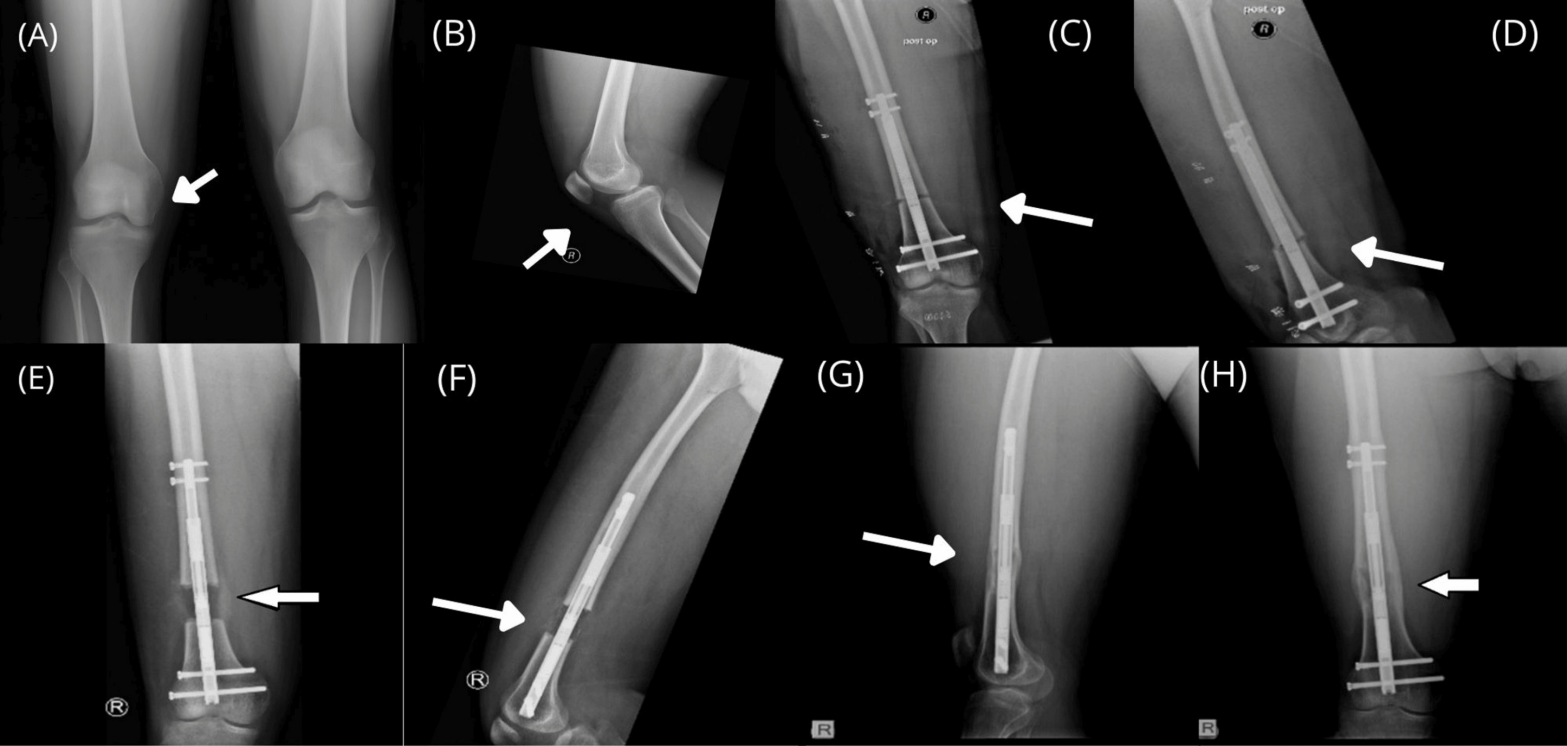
Radiographs Radiographs of a 24-year-old patient. A) Preoperative bilateral AP view, B) preoperative lateral view, C) immediate postoperative AP view, D) immediate postoperative lateral view, E) during lengthening AP view, F) during lengthening lateral view, G) AP view after healing of the lengthening site, H) lateral view after healing of the lengthening site.
